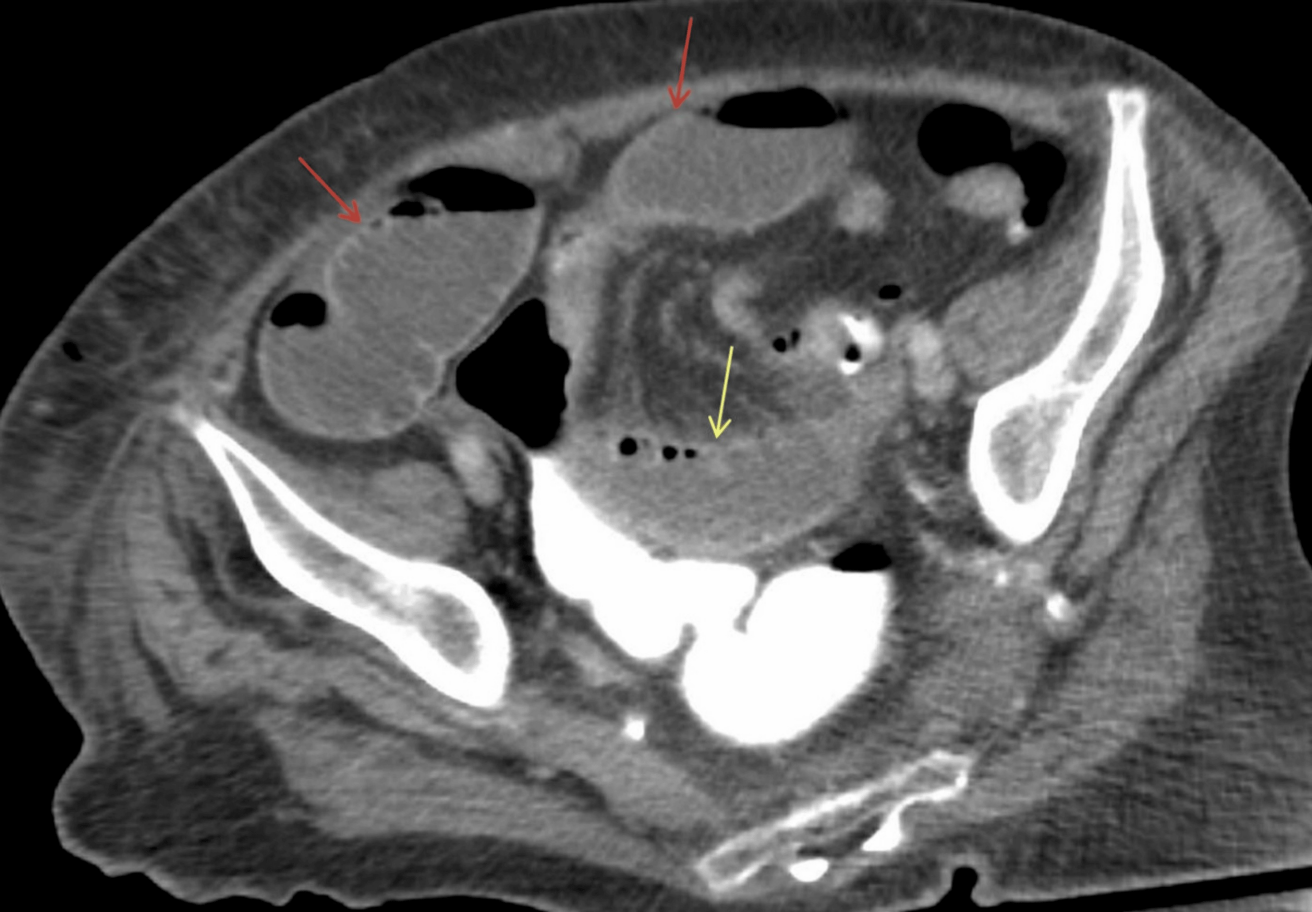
CT abdomen and pelvis with IV contrast (axial view) demonstrates dilated loops of small bowel along the anterior abdominal wall (red arrows) extending into the pelvis (yellow arrow), without evidence of any definite free air. CT: computed tomography; IV: intravenous
